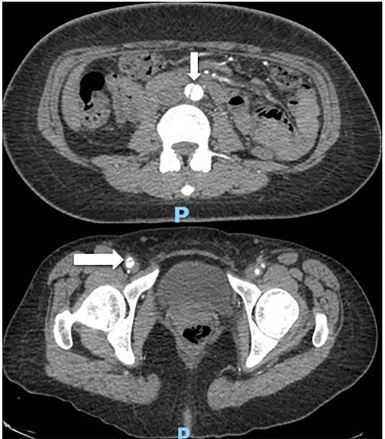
Computed tomography angiogram demonstrating flap in mid-aorta (top arrow) and flap in right common femoral artery (bottom arrow).
